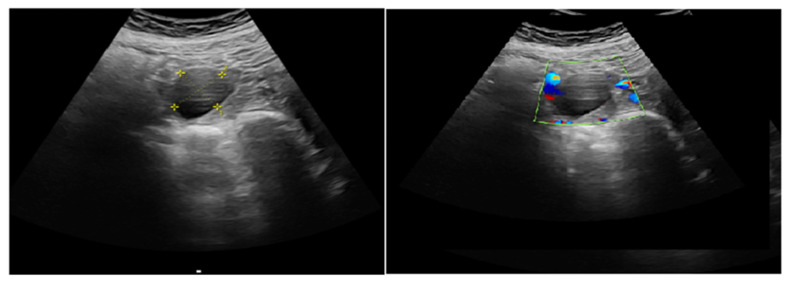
Corpus luteum ultrasound image and color Doppler image.
